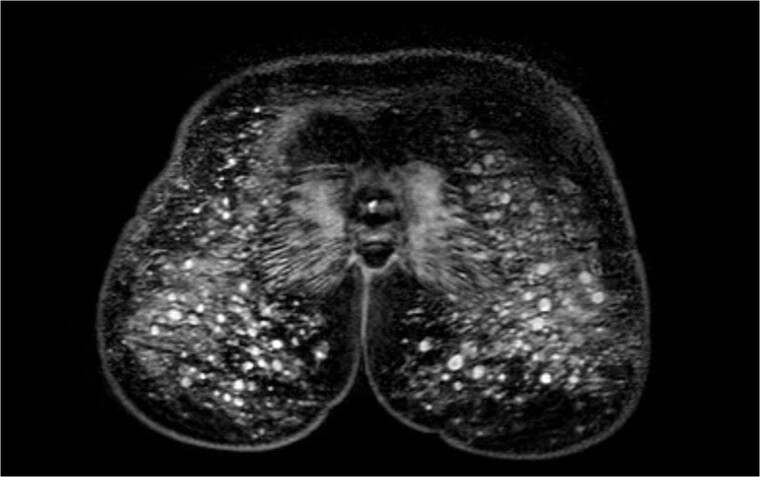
MRI findings: Innumerable rounded lobular intermediate- to low-T1 and intermediate- to high-T2 globules in this patient with ASIA syndrome (autoimmune/inflammatory syndrome induced by adjuvants)
